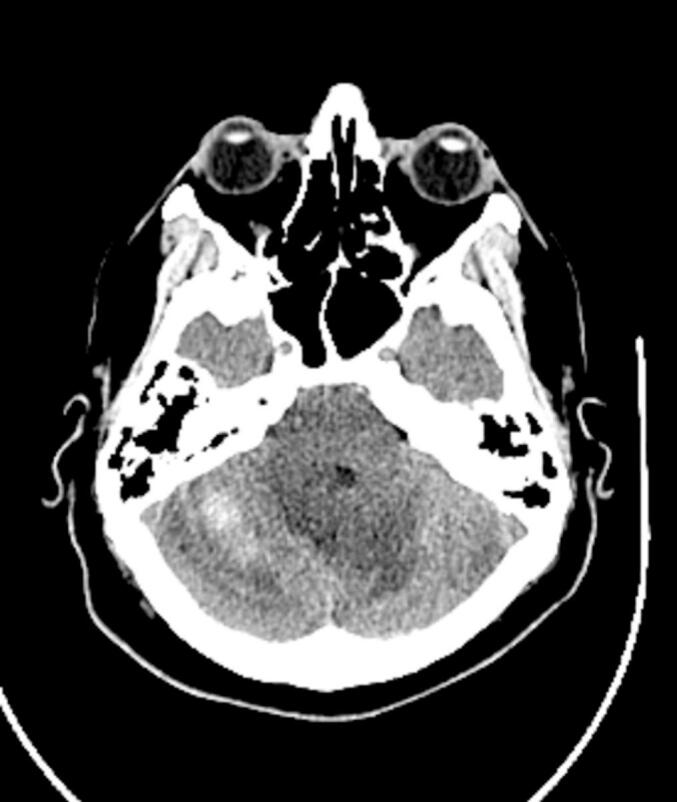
Head CT without IV contrast POD15 demonstrating improvement of right cerebellar hemorrhage during inpatient course.
